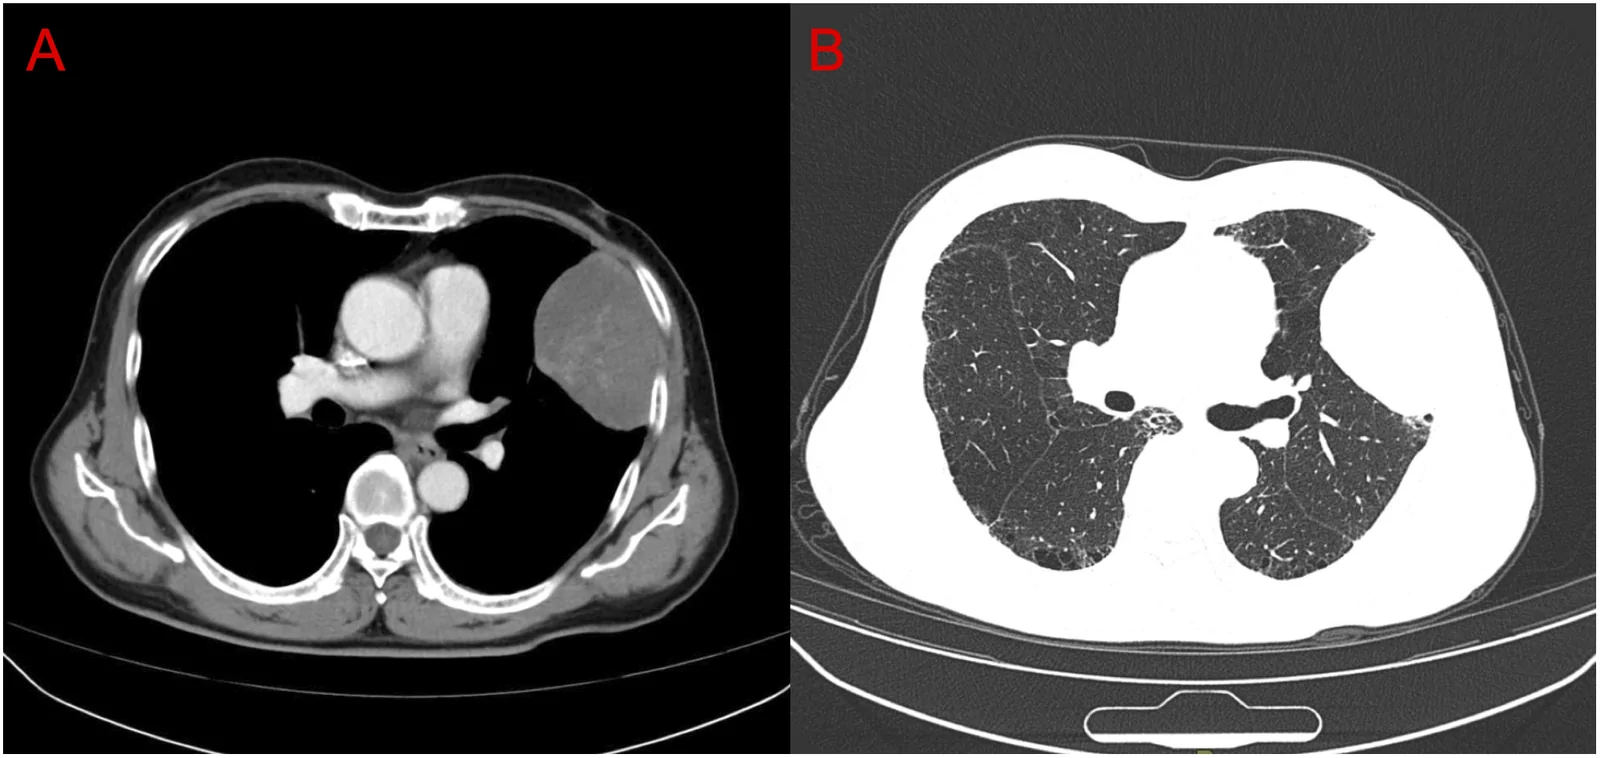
Chest enhanced CT demonstrated a heterogeneous mass in the left upper lobe before surgery. (A) Soft tissue window. (B) Lung window.
